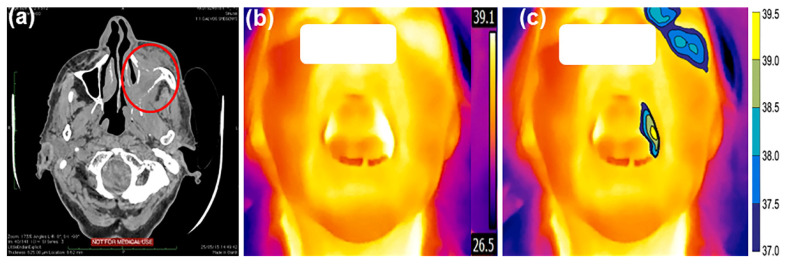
Mapping of a CT-scan image with TI without preprocessing and after segmentation. A CT-scan image (a) is presented in the axial plane and shows large infiltrative pathologic masses on the left side (marked by an oval ellipse). The TI of the same patient is presented in an open mouth position, and the area with higher temperature could be seen on the left side of the mouth cavity before (b) and after the segmentation (c) of images. The corresponding temperature scale bar is given on the right side in °C.
